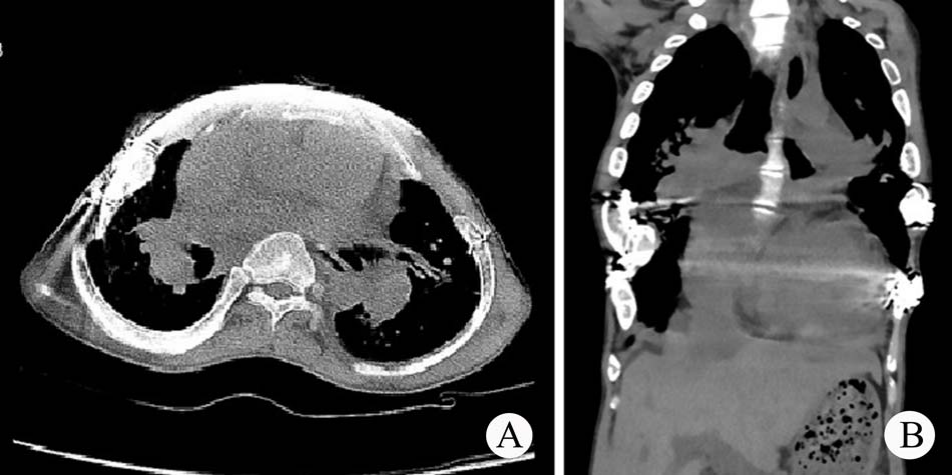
(A) and (B) Postoperative CT scan of thorax.
